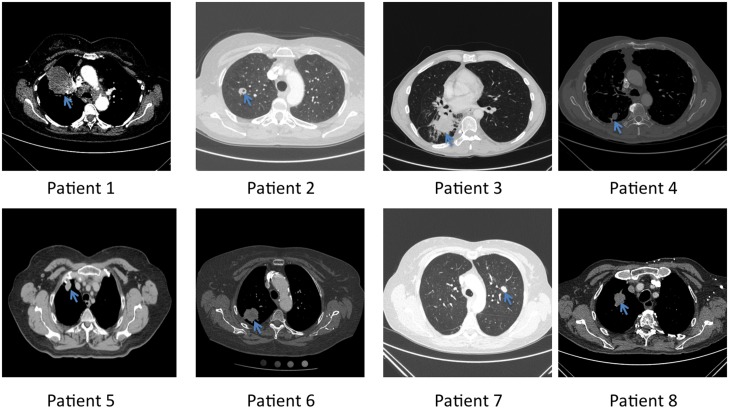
Patients’ computed tomography (CT) scans. CT scans show representative slices of the lung lesions (blue arrow) prior to ENB. Patient numbers correspond to those listed in Table 1.
